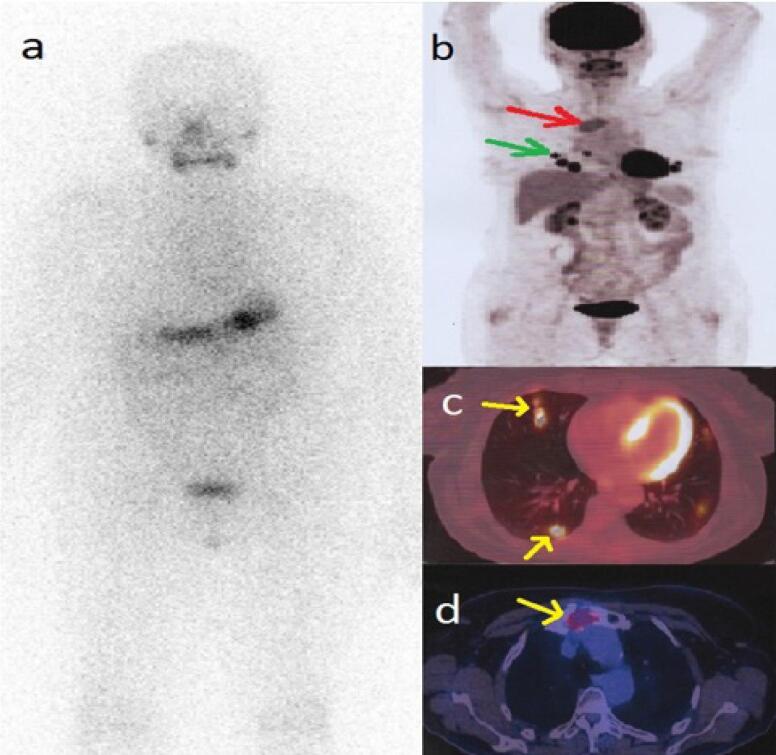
whole-body iodine scan ( a ) after administration of 200 mCi I-131 was negative. FDG PET/CT maximum intensity projection (MIP) ( b ) shows sternal and pulmonary metastases, which are more precisely localized on transaxial slices ( c , d ).
